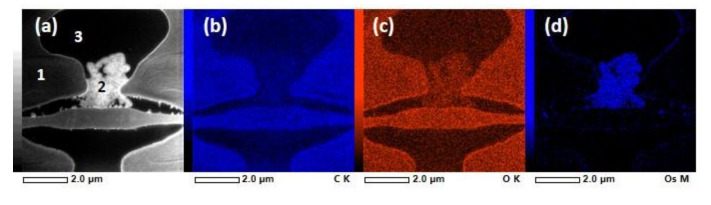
STEM-EDS of resin-embedded knotwood tracheid with some deposit material. (a) STEM image with region numbers corresponding to Table 4. EDS images showing (b) C K α, (c) O K α and (d) Os M α X-ray emission.
